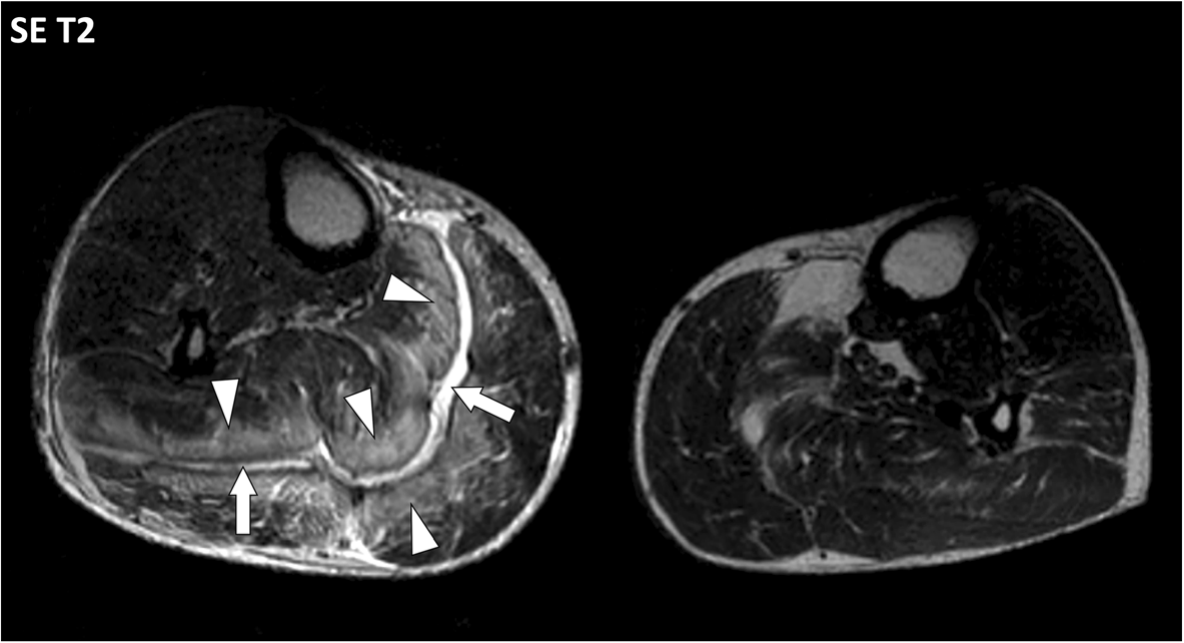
Axial SE T2w image of the legs of a 71-year-old male with necrotizing fasciitis of the right leg. MRI demonstrates thickening of the deep intermuscular fascia between gastrocnemius and soleus muscles with fluid-like signal (arrows) and extensive infiltration of the connective tissue of the adjacent muscles (arrowheads)
